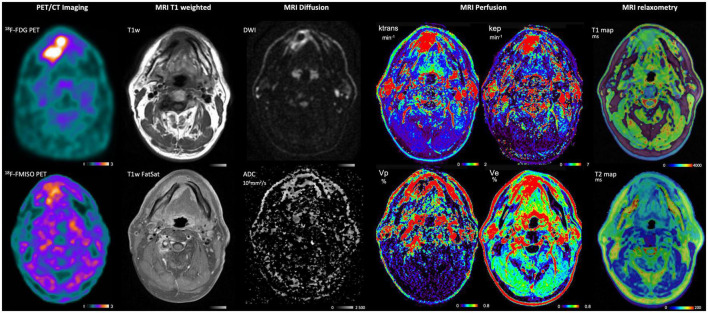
PET and MRI imaging of a patient with HNC included in the study “RTEP8”. Multimodality imaging has been performed with [^18^F]-FDG (metabolism), [^18^F]-FMISO (hypoxia) for the PET imaging. MRI T1 weighted, MRI diffusion, MRI Perfusion and MRI relaxometry have been performed at the same time. This patient had a lesion of the right lower paramedian gum. The T1-weighted sequence showed a hyposignal infiltration and a hypersignal of the lesion with fat saturation. The lesion was in diffusion hypersignal with restriction of free water diffusion. Perfusion sequence Vp showed a contrast within the lesion proving a lack of vascularization in the tumor and might be correlated with the [^18^F]-FMISO uptake. T1 and T2 relaxation time velocities are decreased within the lesion.
